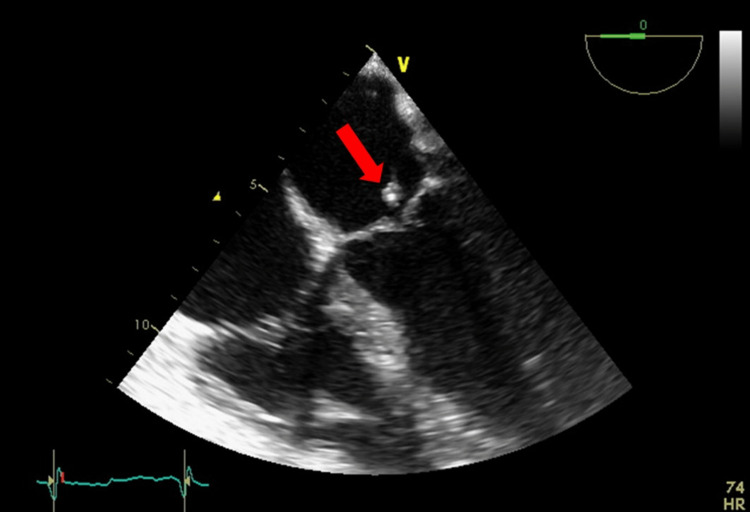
Four chamber view on trans-esophageal echocardiogram (TEE) showing mitral valve vegetation (red arrow). TEE: trans-esophageal echocardiogram
